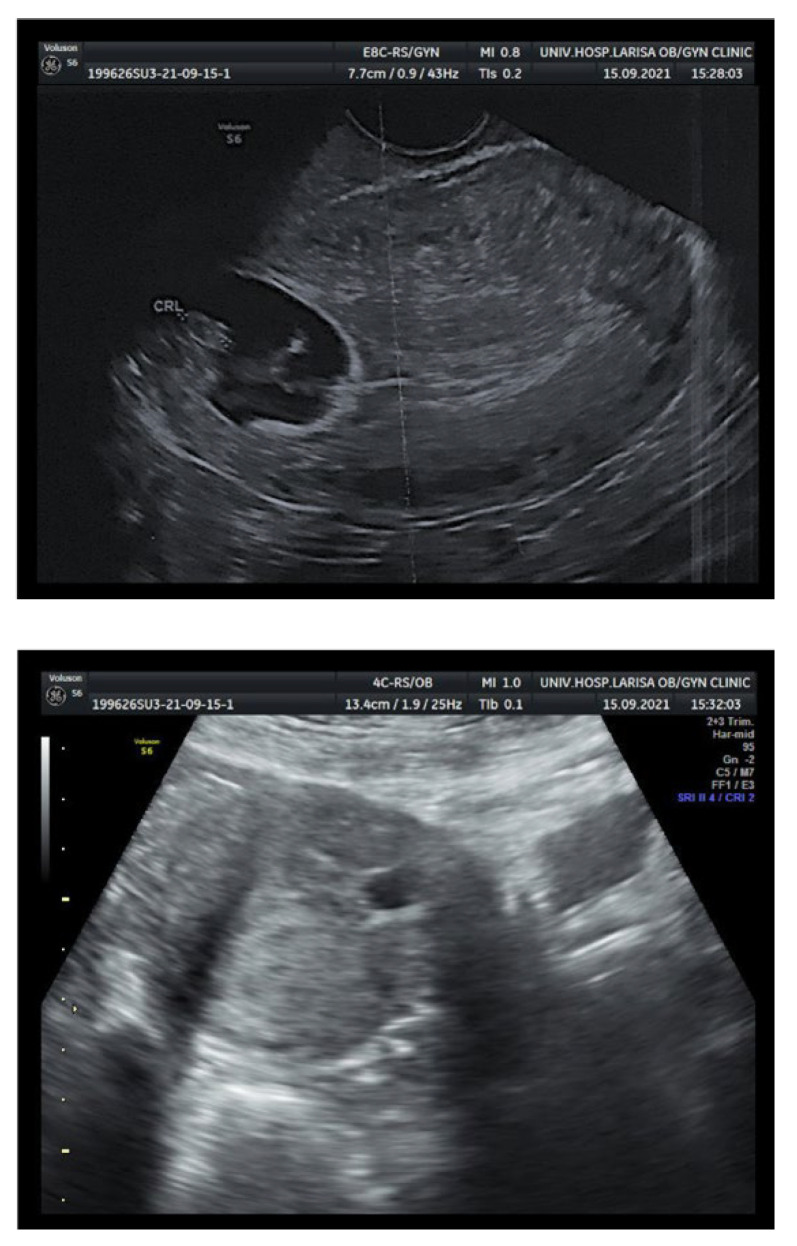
Transvaginal ultrasound showing the gestational sac attached to the caesarean scar.
